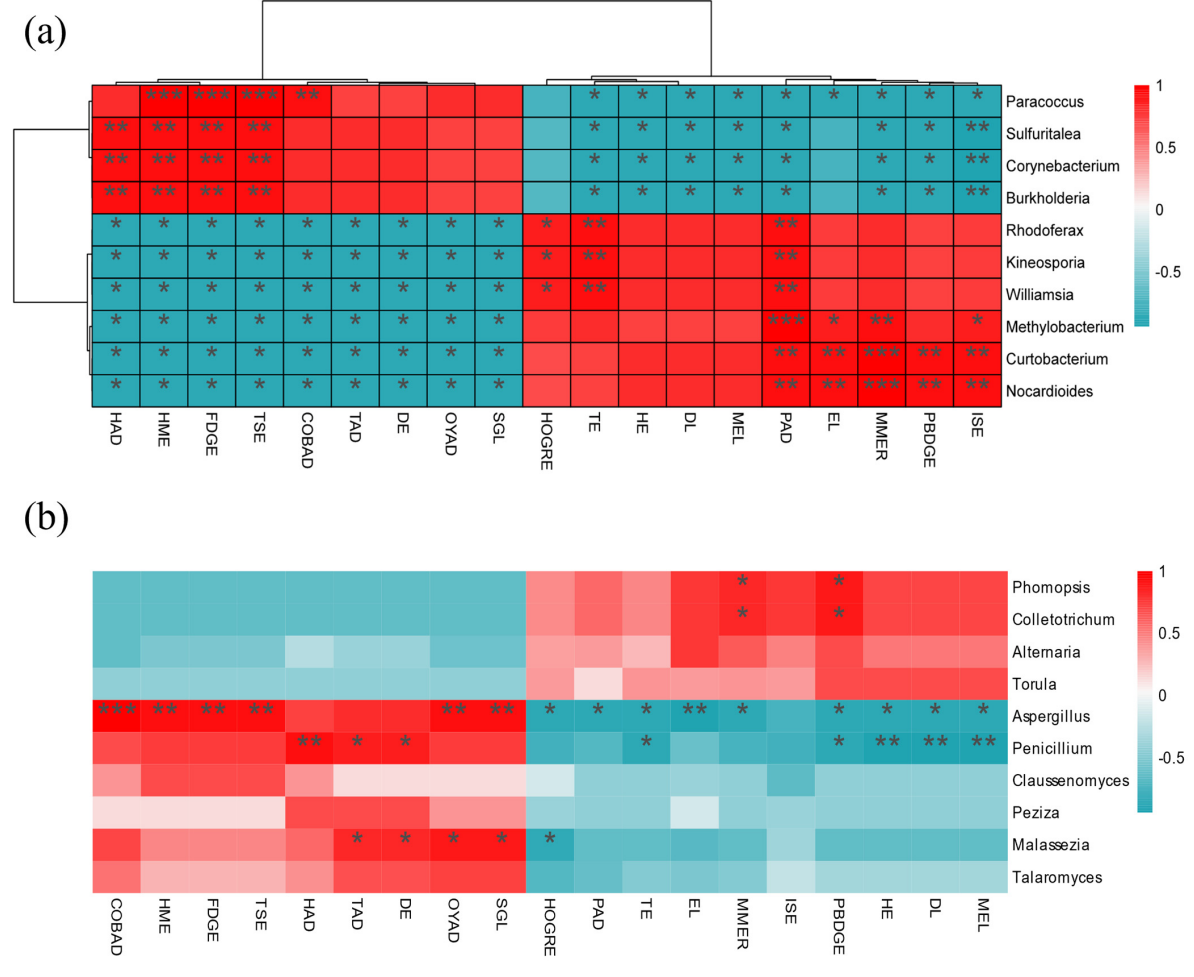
Association analysis of leaf microorganisms and metabolome (a) bacteria, (b) fungi. MEL: 2-Methoxy-4-ethenylphenol; ISE: Idesin salicylate; HOGRE: 2-Hydroxyphenol-1-O-glucosyl(6→1)rhamnoside; LMER: L-Methionine methyl ester; HE: 8-Hydroxyguanosine; TE: Thymine; FDGE: Furanofructosyl-α-D-(6-mustard acyl)glucoside; COBAD: 3-[(1-Carboxyvinyl)oxy]benzoic acid; TAD: 2,3,4-Trihydroxybenzoic acid; DE: 2′-Deoxycytidine; TSE: Tartronate semialdehyde; HME: 4-Hydroxy-3-methoxymandelate; HAD:β-Hydroxyisovaleric acid; OYAD: 9,12-Octadecadien-6-Ynoic Acid; DGL:1-Stearidonoyl-Glycerol. The single asterisk (*) represents the significance of *p* < 0.05, the double asterisk () represents *p* < 0.01, and the triple asterisk (*) represents *p* < 0.001 value.
